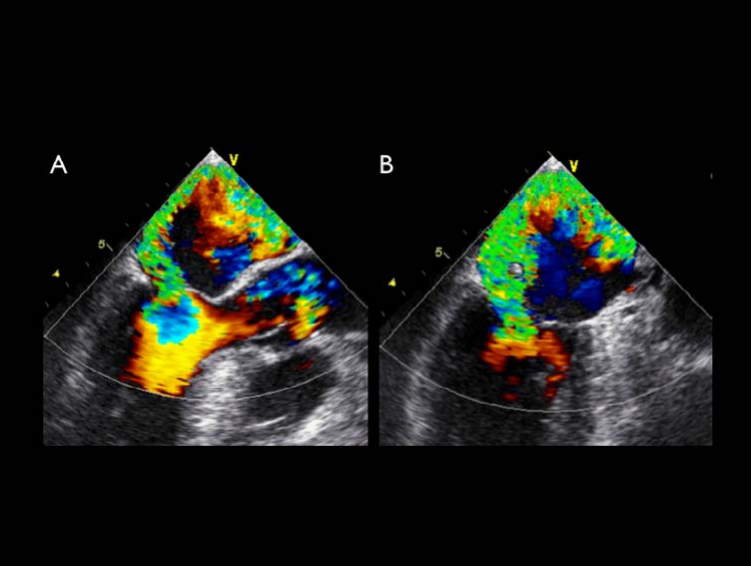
A-B A transesophageal echocardiographic long axis and two chamber midesophageal view with color Doppler demonstrating an eccentric posteriorly directed jet of severe mitral regurgitation.
